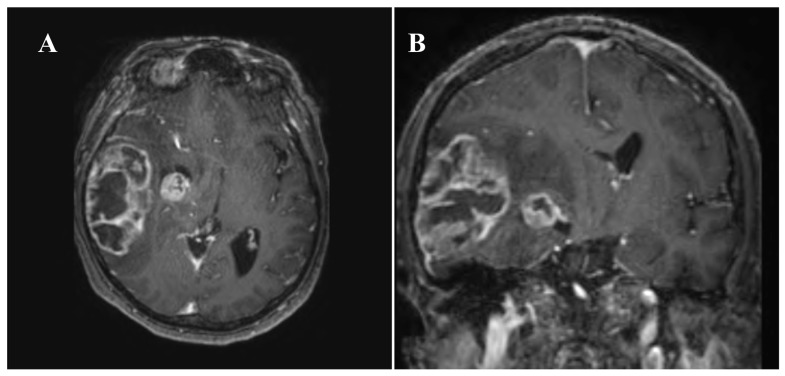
Preoperative T1-weighted brain MRI axial (A) and coronal (B) sequences with gadolinium reveal the presence of lesions with irregular margins, containing diffuse necrotic and hemorrhagic/hemosiderin-rich intralesional components. It exhibits pathological and heterogeneous contrast enhancement, localized in the right lateral temporal region. This lesion measures approximately 70 mm antero-posteriorly, 40 mm medio-laterally, and 56 mm cranio-caudally. It is associated with a substantial perifocal edema extending into the temporal, deep frontoparietal, and ipsilateral nucleus-capsule. Another smaller lesion of similar significance and characteristics, with a rounded morphology, is observed in the ipsilateral temporo-mesial region.
